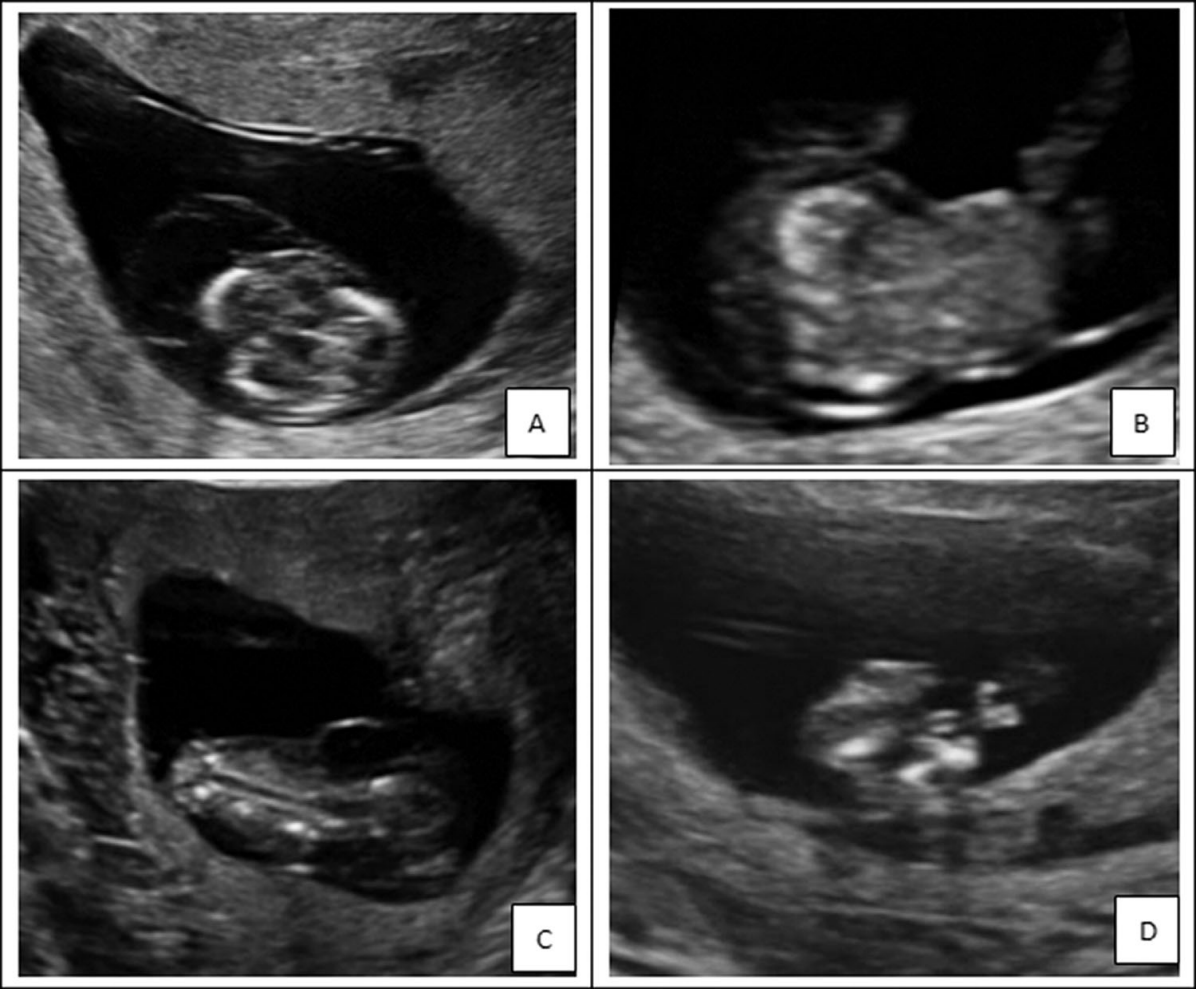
The ultrasound examination of the case with diastrophic dysplasia. The ultrasound examination showed that cystic hygroma (A), omphalocele (B), spinal malformation (C) and short limbs (D).
